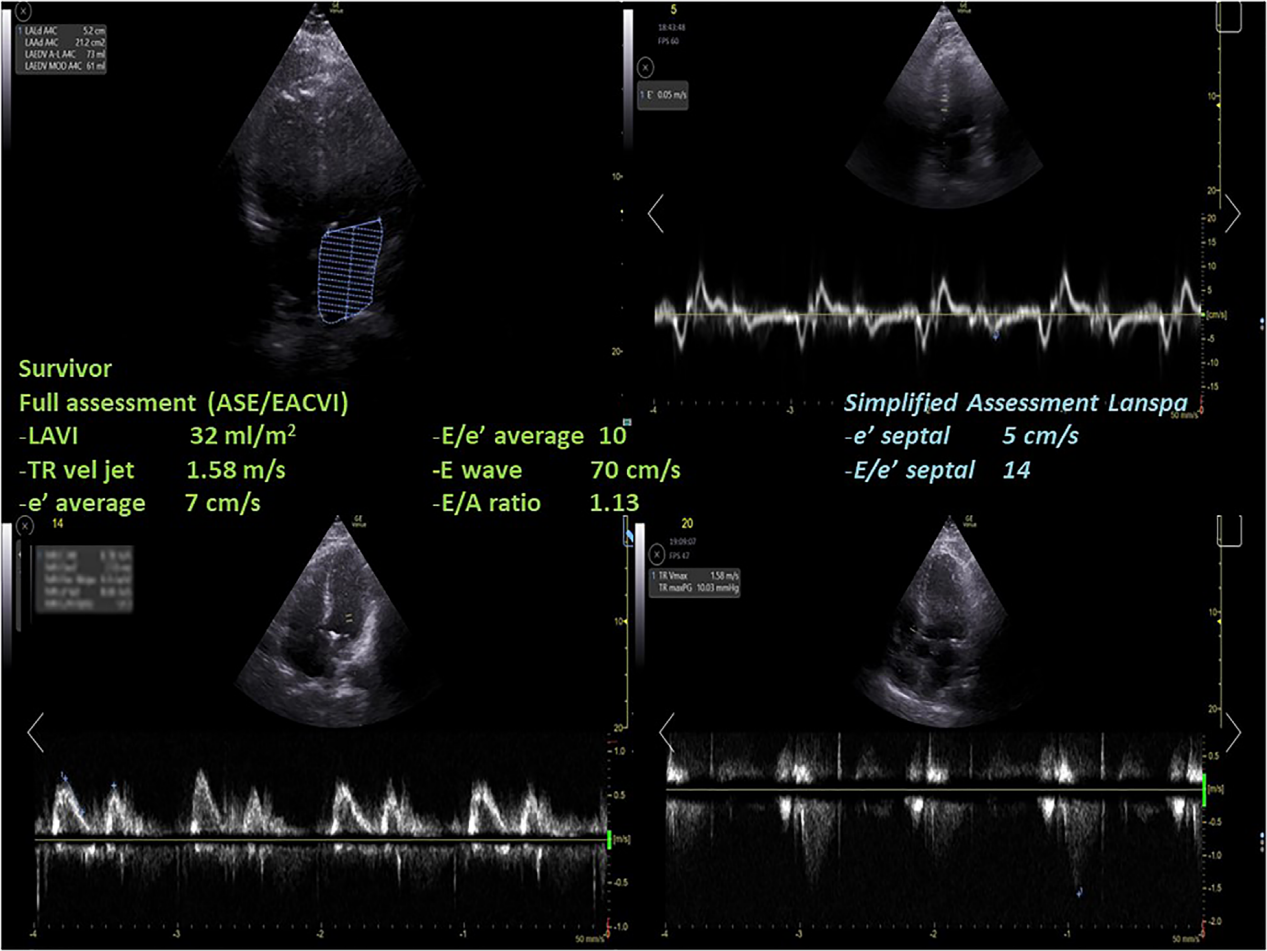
A case of a survivor where the assessment with full criteria resulted in a normal left ventricular diastolic function, while using the simplified assessment according to Lanspa criteria a diagnosis of grade III left ventricular diastolic dysfunction was made. ASE, American Society of Echocardiography; EACVI, European Association of Cardiovascular Imaging; LAVI, left atrial volume index; TR, tricuspid regurgitation.
